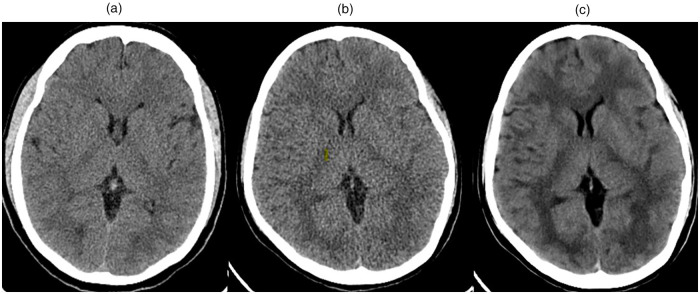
Axial CT image of a 58-year old man with (a) standard-dose-iDose^4^ and axial CT image of a 59-year-old man with (c) low-dose-DLIR showing better delineation of the gray and white matter compared with low-dose-iDose^4^
